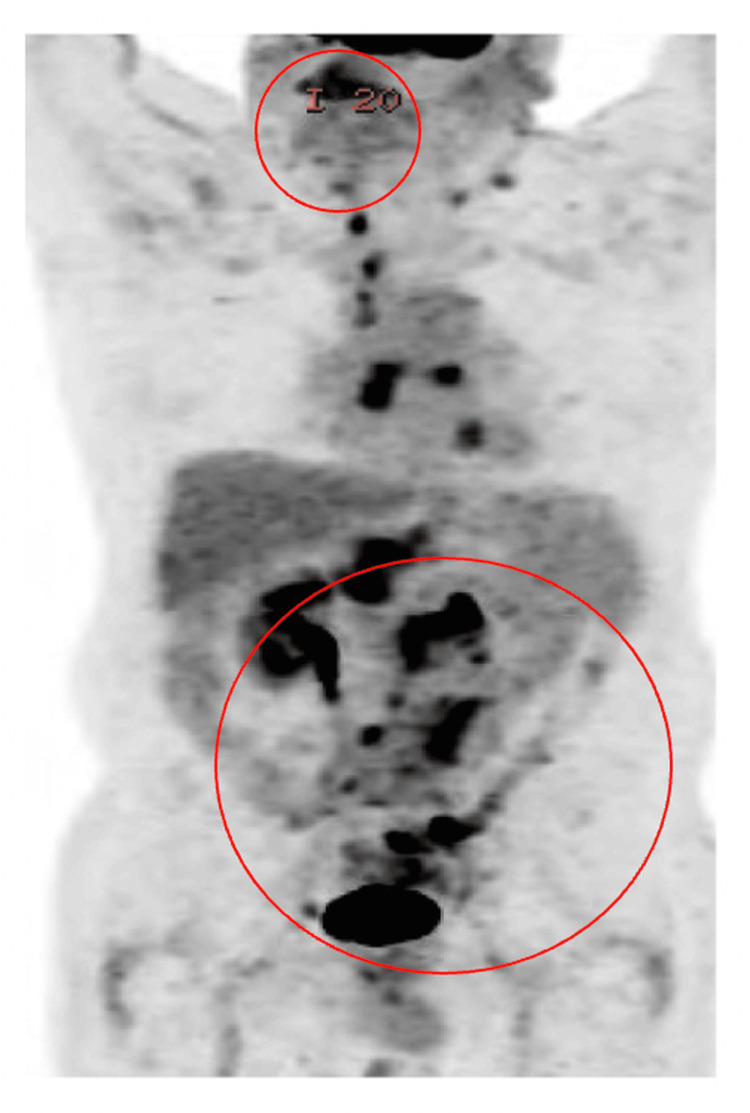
PET scan showing extensive fluorodeoxyglucose (FDG)-avid bilateral lymphadenopathy involving the lower cervical, mediastinal, retrocrural, and retroperitoneal regions (circles indicate hypermetabolic lymph nodes).
